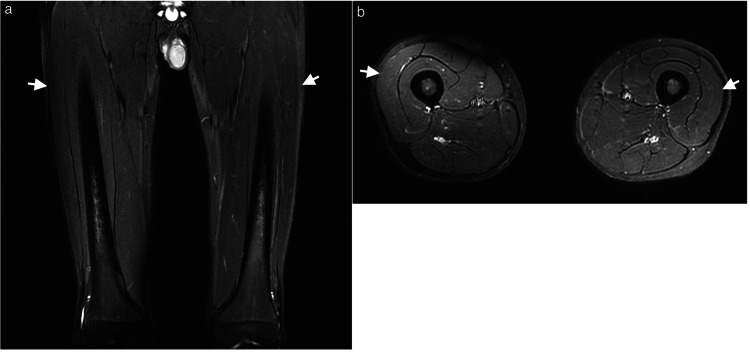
MRI findings on IST Day 50 (18 days after CsA discontinuation) (a) Short tau inversion recovery (STIR) coronal, (b) STIR axial The STIR high signal areas in both thighs had disappeared (white arrows). IST: immunosuppressive therapy; CsA: cyclosporine
